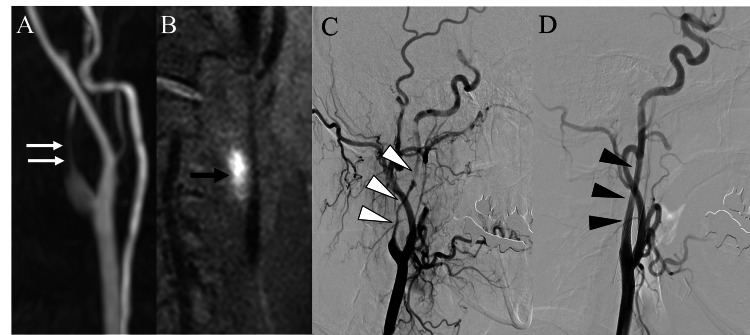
Radiological images at the second presentation. A: Cervical MRA showing near occlusion of the right ICA (white arrows). B: T1-weighted black-blood MRI demonstrating a high signal intensity area in the right ICA, consistent with an intimal hematoma (black arrow). C: Preoperative DSA showing severe stenosis of the right ICA (white arrowheads). D: Postoperative DSA demonstrating successful CAS with restored patency of the right ICA (black arrowheads). ICA: internal carotid artery
